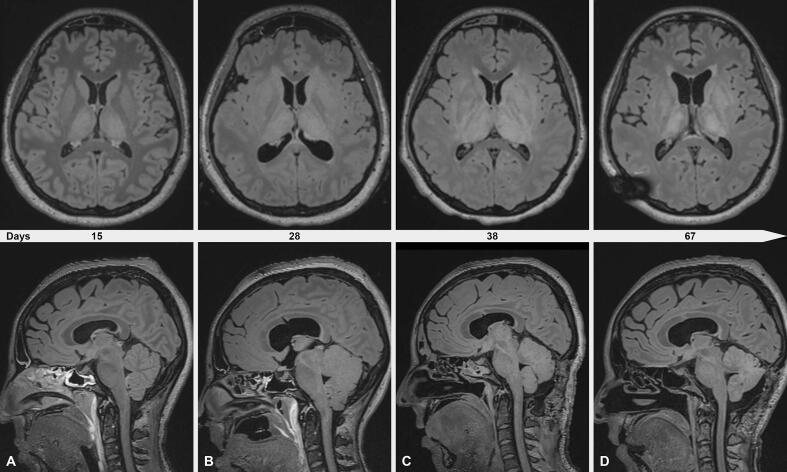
Longitudinal magnetic resonance imaging. For comparability, all transverse sections were taken at the level of the splenium corpori callosi and all sagittal sections were taken slightly lateral to the midline. A) Bilateral mesodiencephalic inflammatory T2/FLAIR-hyperintensities typical of TBE on day 15. B) Progression of the aforementioned findings on day 28. Furthermore, newly developed hydrocephalus despite placement of an external ventricular drain and generalized infratentorial edema. C) Further progression of the aforementioned findings on day 38. Regression of the infratentorial edema after bilateral suboccipital craniectomy in the meantime. D) Regression of supra- and infratentorial inflammatory findings on day 67. Meanwhile, a ventriculoperitoneal shunt was placed (note the artifact in the right parietal region).
